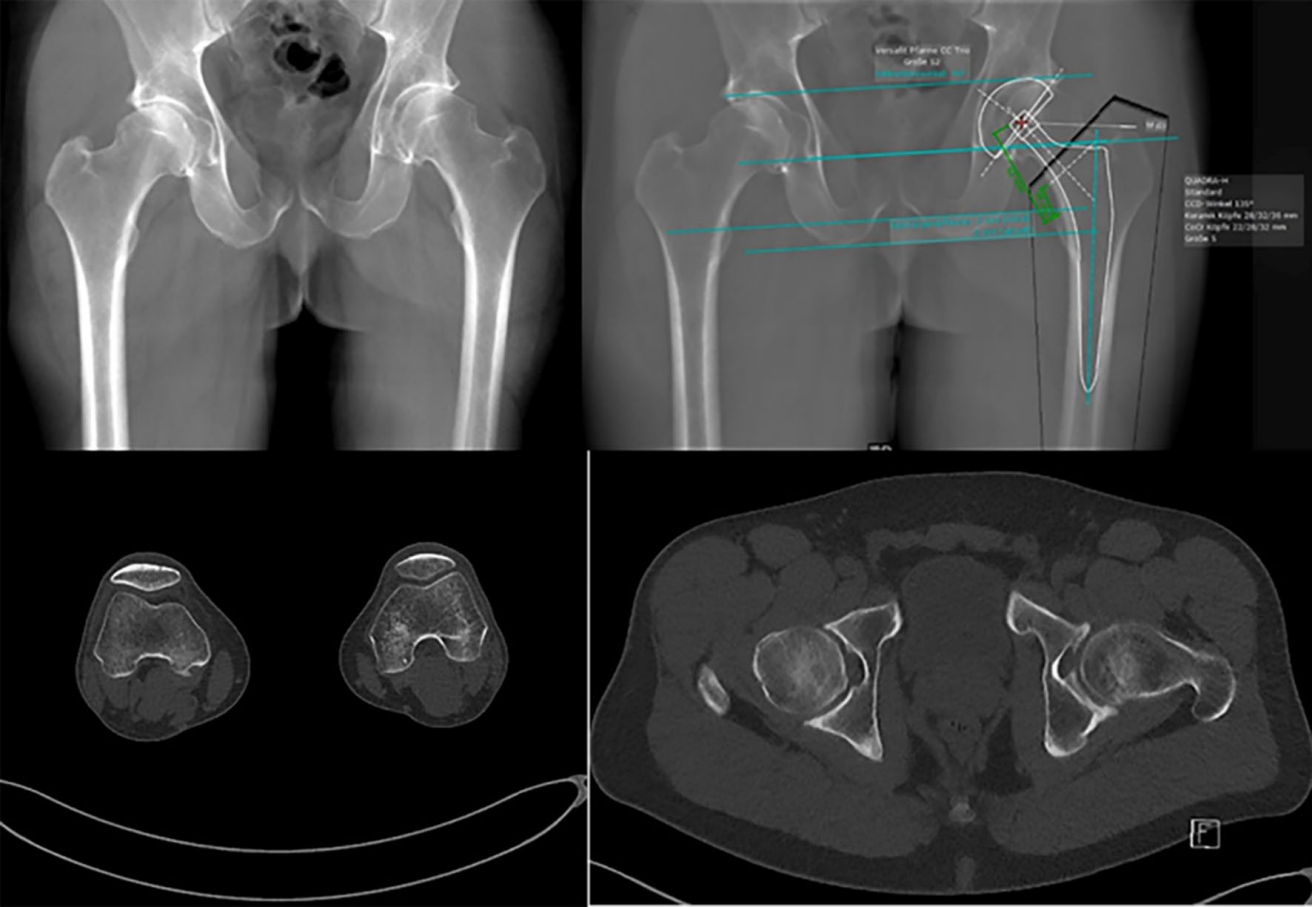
Top left*: Radiograph-like image reconstructed from CT image data. *Top right*: THA templating performed on radiograph-like image. *Bottom left*: Rotation of femoral condyle. *Bottom right*: Rotation of femoral neck
